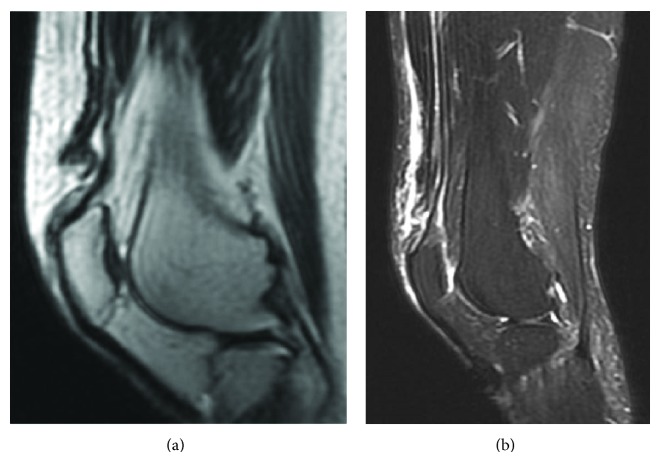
Preoperative MRI image in Case  2. (a) A sagittal proton density-weighted image showing maintained continuity of the deep layer of the quadriceps tendon and patella, in addition to disruption and deflection of the superficial layer. (b) Short tau inversion recovery (STIR) imaging showing the same changes as in (a).
